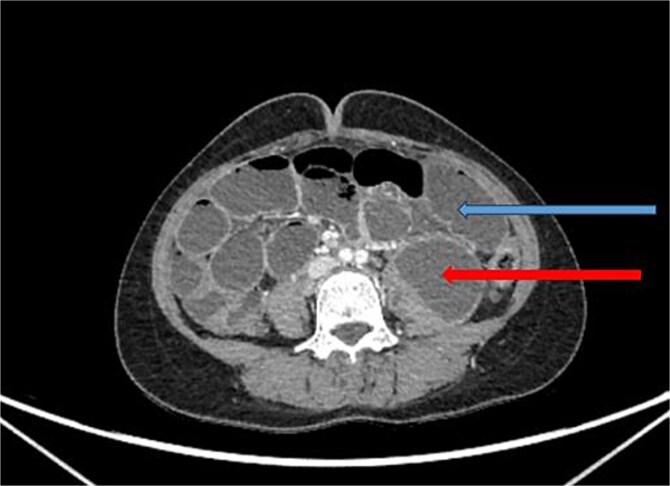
CT abdomen/pelvis with contrast, upper arrow showing dilated small bowel loops and lower arrow showing collection in left psoas muscle.
